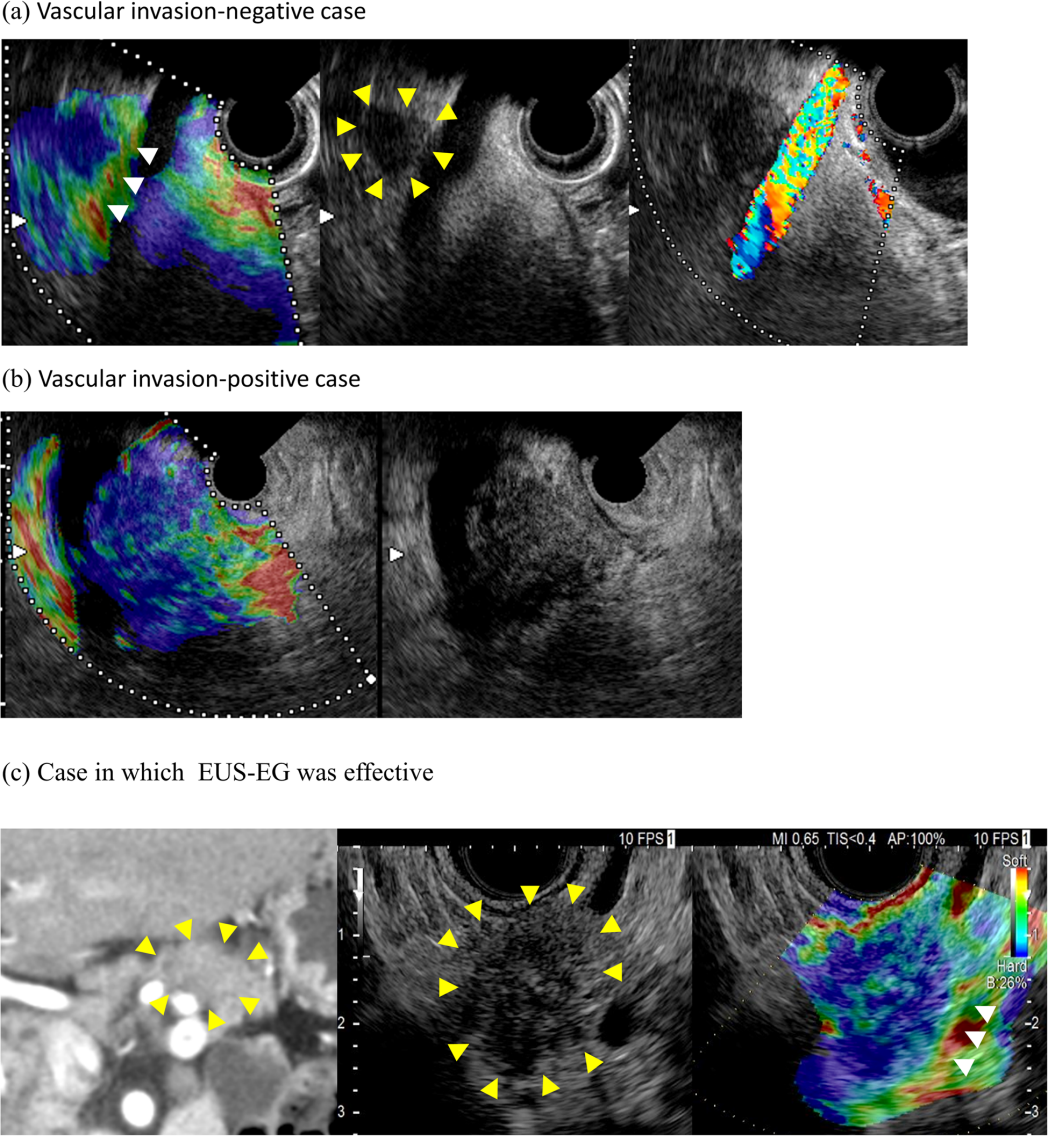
Diagnosis of vascular invasion by Endoscopic ultrasonography Elastography (EUS-EG). a Vascular invasion-negative case. Starting from the left: EUS-EG, EUS B-mode and color Doppler. In B-mode, vascular invasion of the tumor (yellow arrow) is unclear in EUS B-mode image, whereas the colored band (arrow) in EUS-EG image clearly identifies a vascular invasion-negative. b Vascular invasion-positive case. Starting from the left: EUS-EG and EUS-B-mode. In EUS-B-mode, vascular invasion of the tumor is not clear, whereas the absence of a colored band in EUS-EG image permits diagnosis of the site as vascular invasion-positive. c Vascular invasion-negative case in which EUS-EG was effective. Starting from the left: CECT, EUS B-mode and EUS-EG. In CECT, the tumor (yellow arrow) appears to invade the splenic artery. In B-mode, vascular invasion of the tumor (yellow arrow) is unclear in EUS B-mode image, whereas the colored band (arrow) in EUS-EG image clearly identifies a vascular invasion-negative. Pathologically, this case did not infiltrate the splenic artery. CECT: contrast enhanced computed tomography, EUS: endoscopic ultrasonography, EUS-EG: endoscopic ultrasonography elastography
